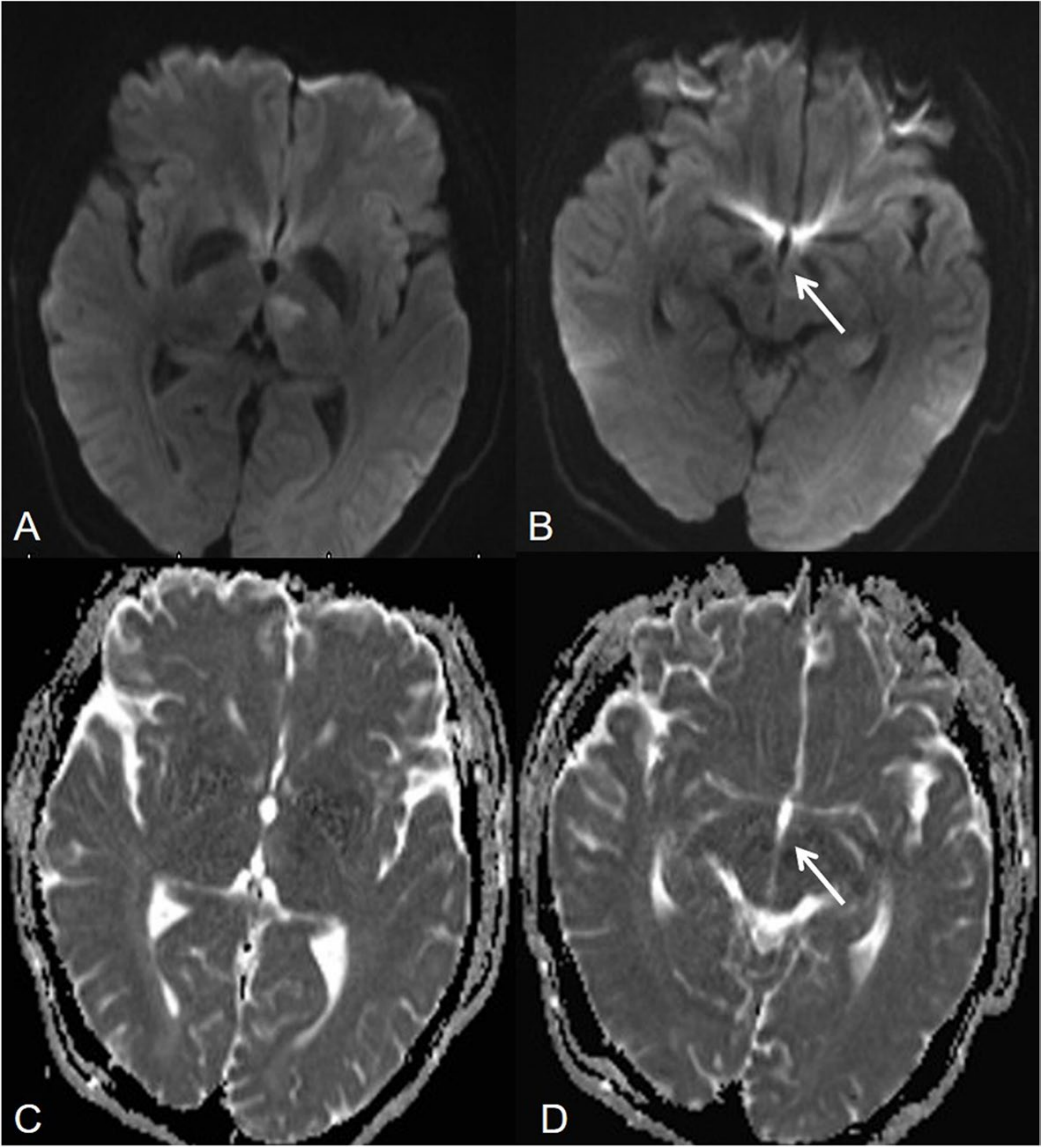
Bilateral paramedian thalamic infarction with left red nucleus. Case 7. Axial DWI and ADC map demonstrate acute symmetrical infarcts in the bilateral paramedian thalami (A, black arrow) and left red nucleus (B, white arrow)
